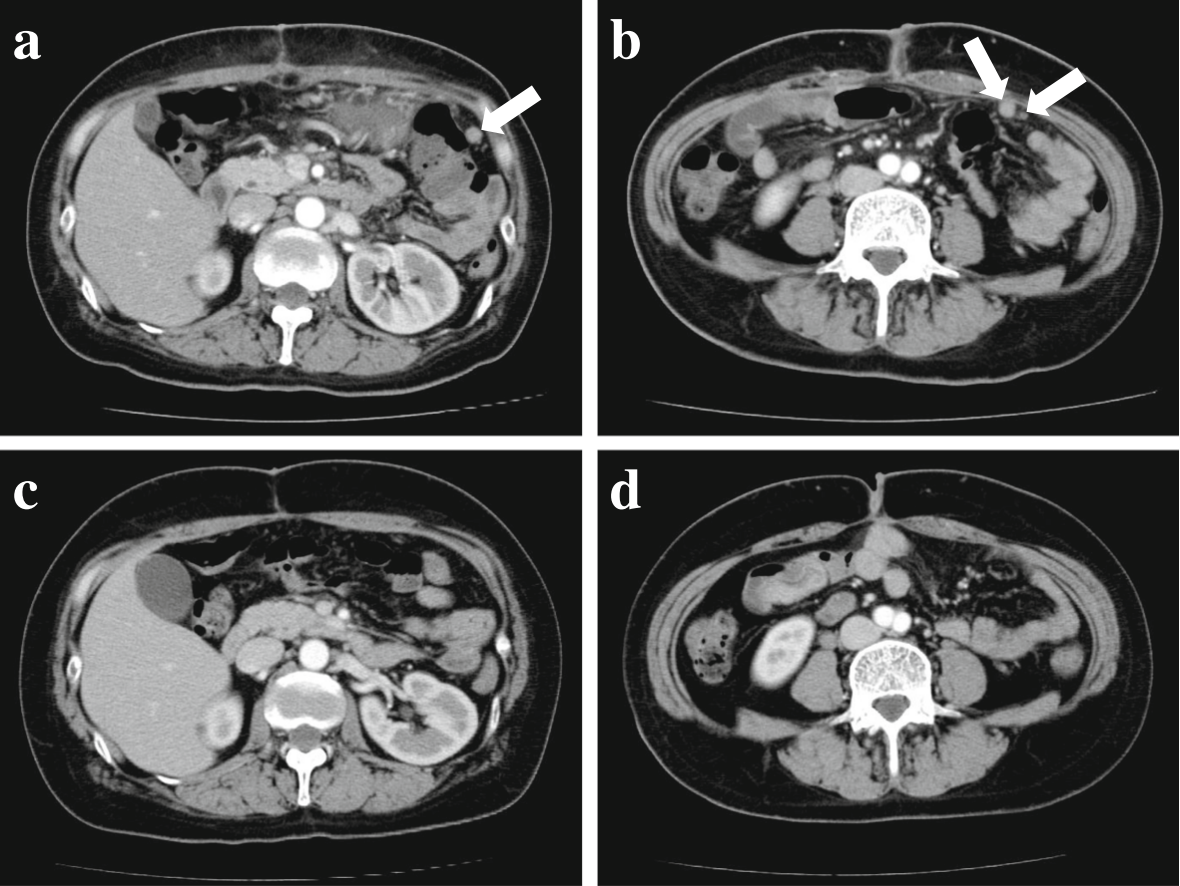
a, b Postoperative computed tomography, before imatinib administration, showing several recognizable disseminated lesions in the abdominal cavity (*arrows*). c, d Follow-up computed tomography, 9 months after imatinib administration, showing ambiguous residual disseminated lesions
